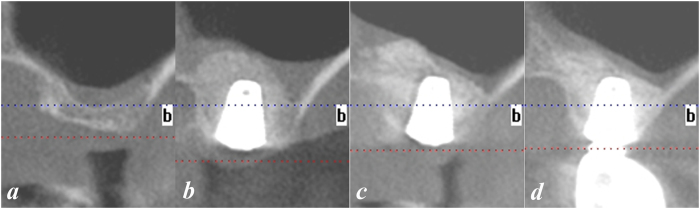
Radiographic measurements. CT scans were obtained before (a), immediately after (b), at 6 months (c) and at 1 year (d) after implant placement.
